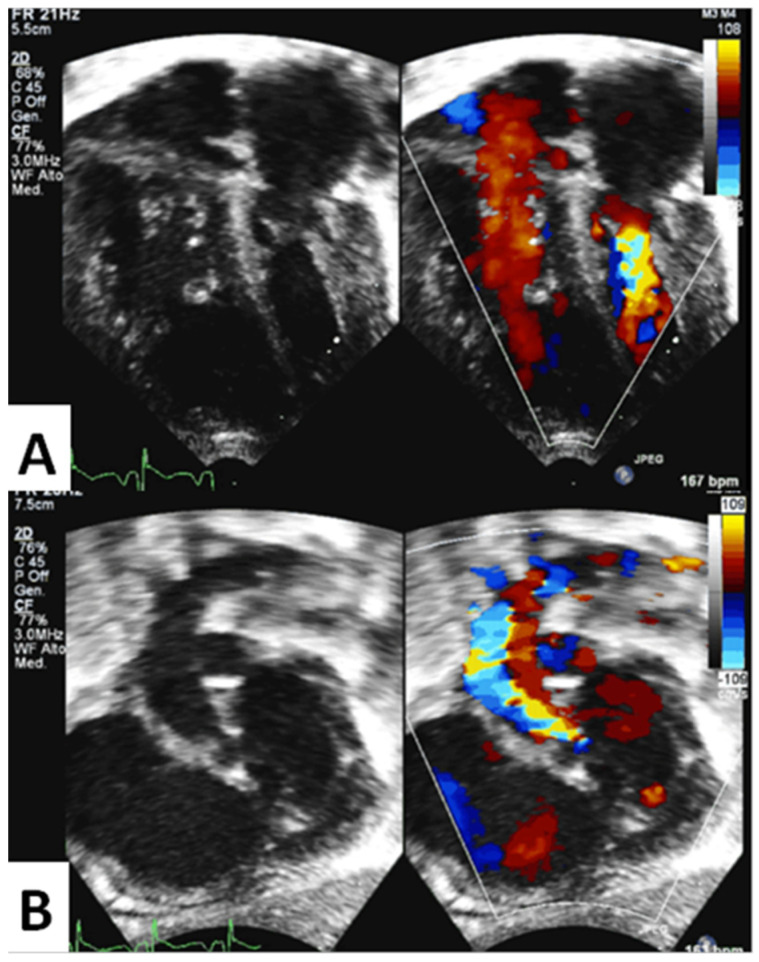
Panel (A). Apical 4−chamber view of a severely underdeveloped left ventricle with mitral annular hypoplasia. Panel (B). Subcostal left oblique view showing severe aortic annular and arch hypoplasia; aortic stenosis is evident with color−Doppler. Antegrade aortic flow is obstructed but still present.
